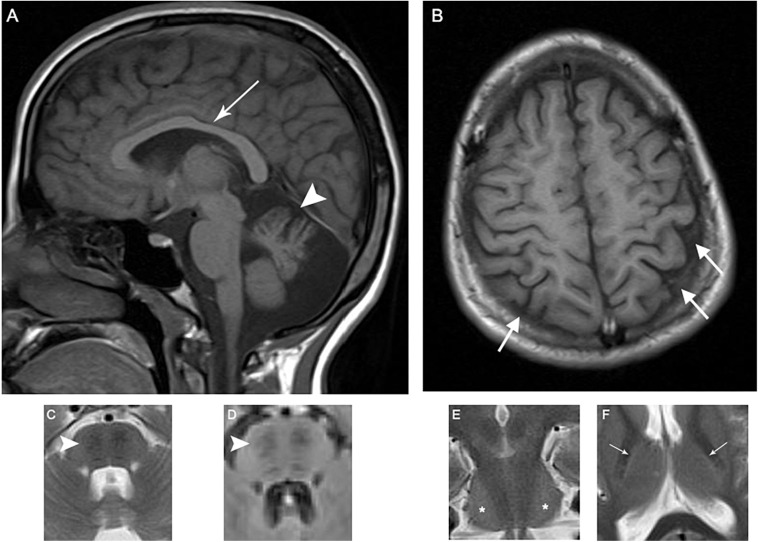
Patient 1 brain MRI revealing the characteristic ARSACS findings. MRI of the index patient reveals atrophy of the superior cerebellar vermis (arrowhead) and thinning of the posterior midbody of corpus callosum (white arrow) (A, T1); bilateral parietal atrophy, mostly prominent on the left side (white arrows) (B, T1); bilateral hypointense stripes in pons (arrowheads) (C, T2; D, FLAIR); bilateral hyperintensity of lateral pons (stars) on coronal images (E, T2); and a hyperintense rim around both thalami (white arrows) (F, T2).
